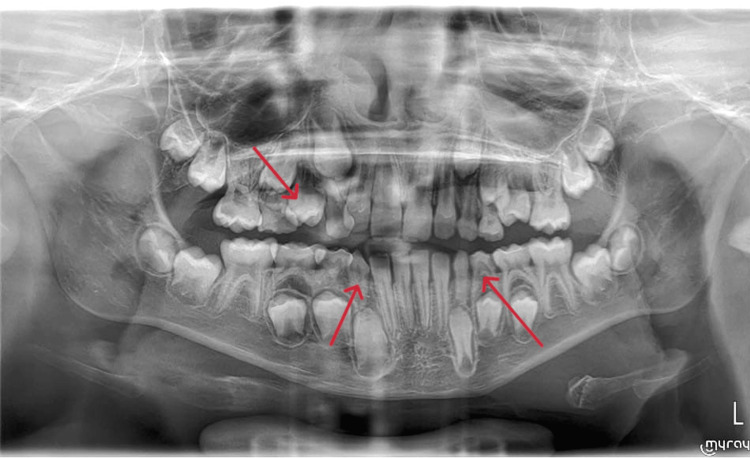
X-ray orthopantomogram showing dental caries with no deep-seated collection being identified (arrows)
